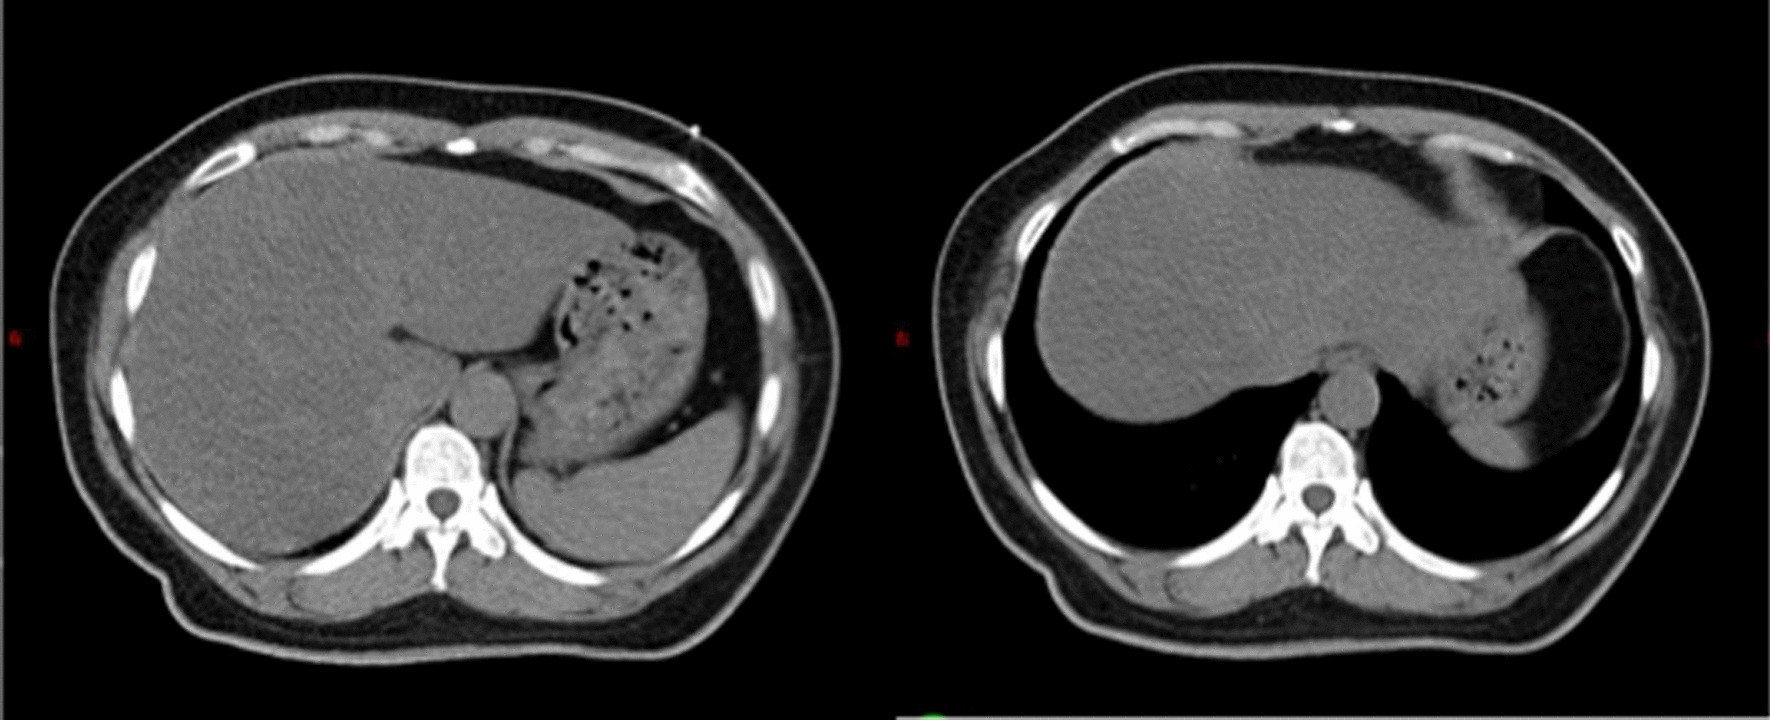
Computed tomography scans for both FB (left) and DIBH (right) at the same axial location in 1 patient. During DIBH, the stomach was pushed downwards and backwards by the left lung, leading to lower dose distribution
